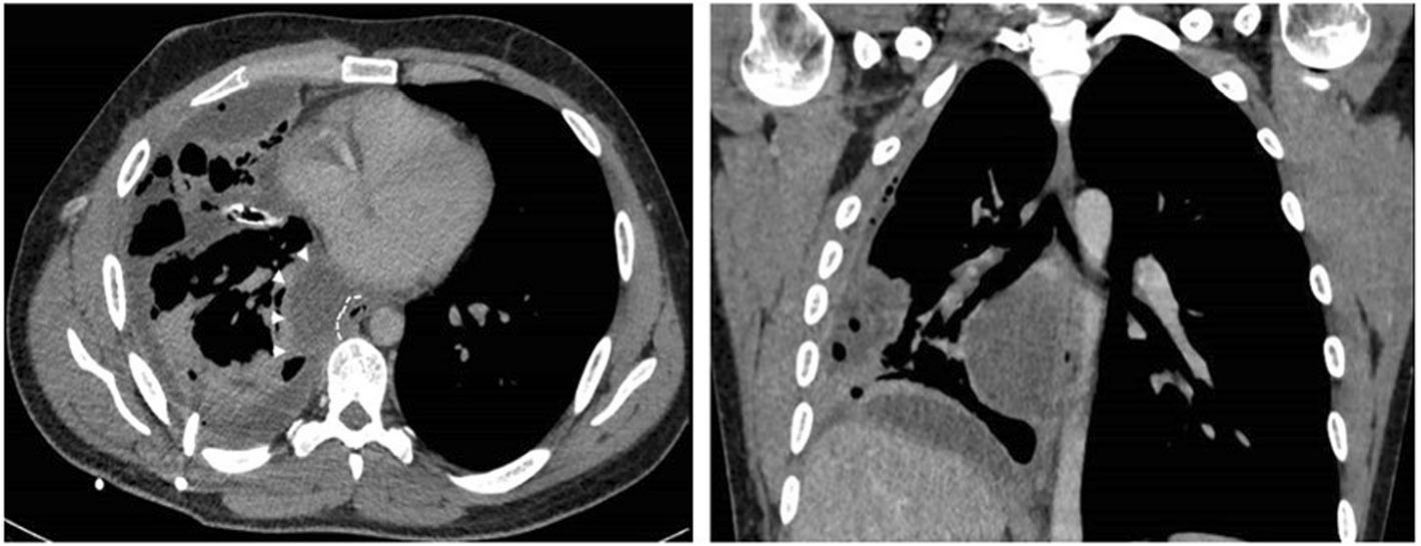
CT scan with a massive pleural effusion in the right hemithorax and complete atelectasis of the ipsilateral lung
